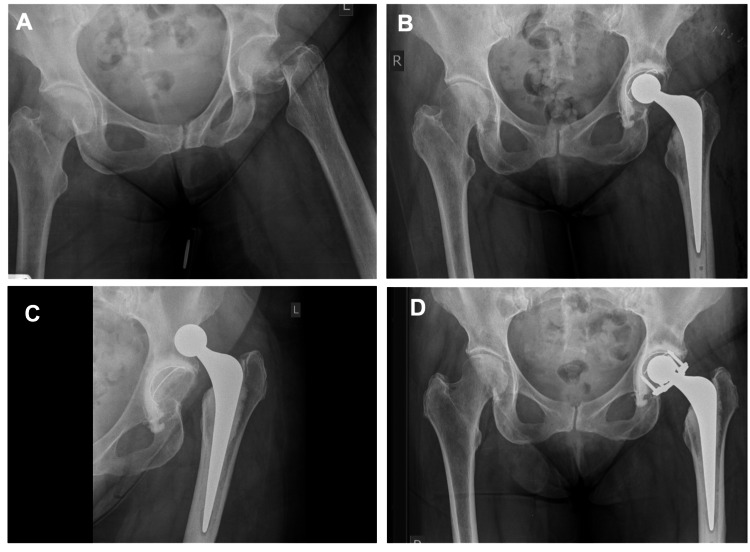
Plain film anteroposterior radiographs in the case of a 72-year-old female A. Left intracapsular fractured neck of femur; B. Cemented total hip arthroplasty (THA); C. Posterior-superior dislocation; D. Reduced THA with posterior lip augmentation device
